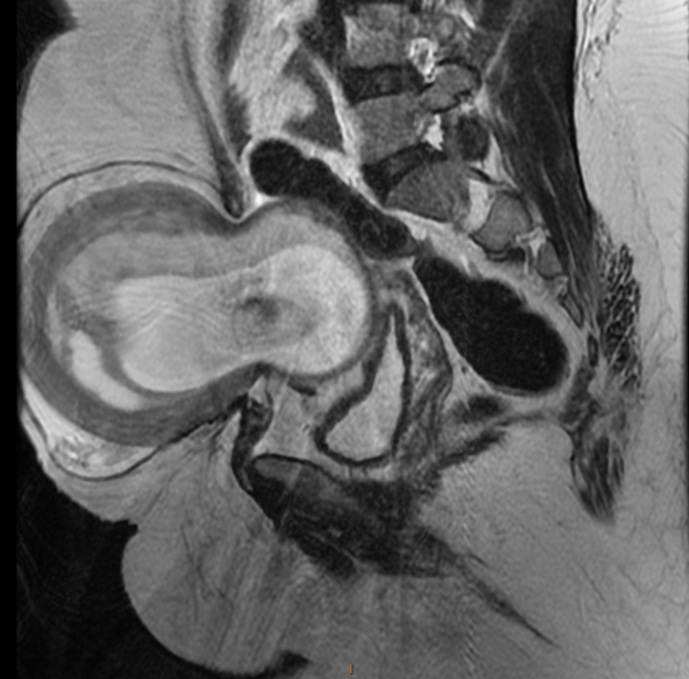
sagittal cut:MRI show strangulation of a pregnant uterus through an orifice of 6 cm, resulting in a strangulated hernia of a pregnant uterus at the umbilical level, with the.
